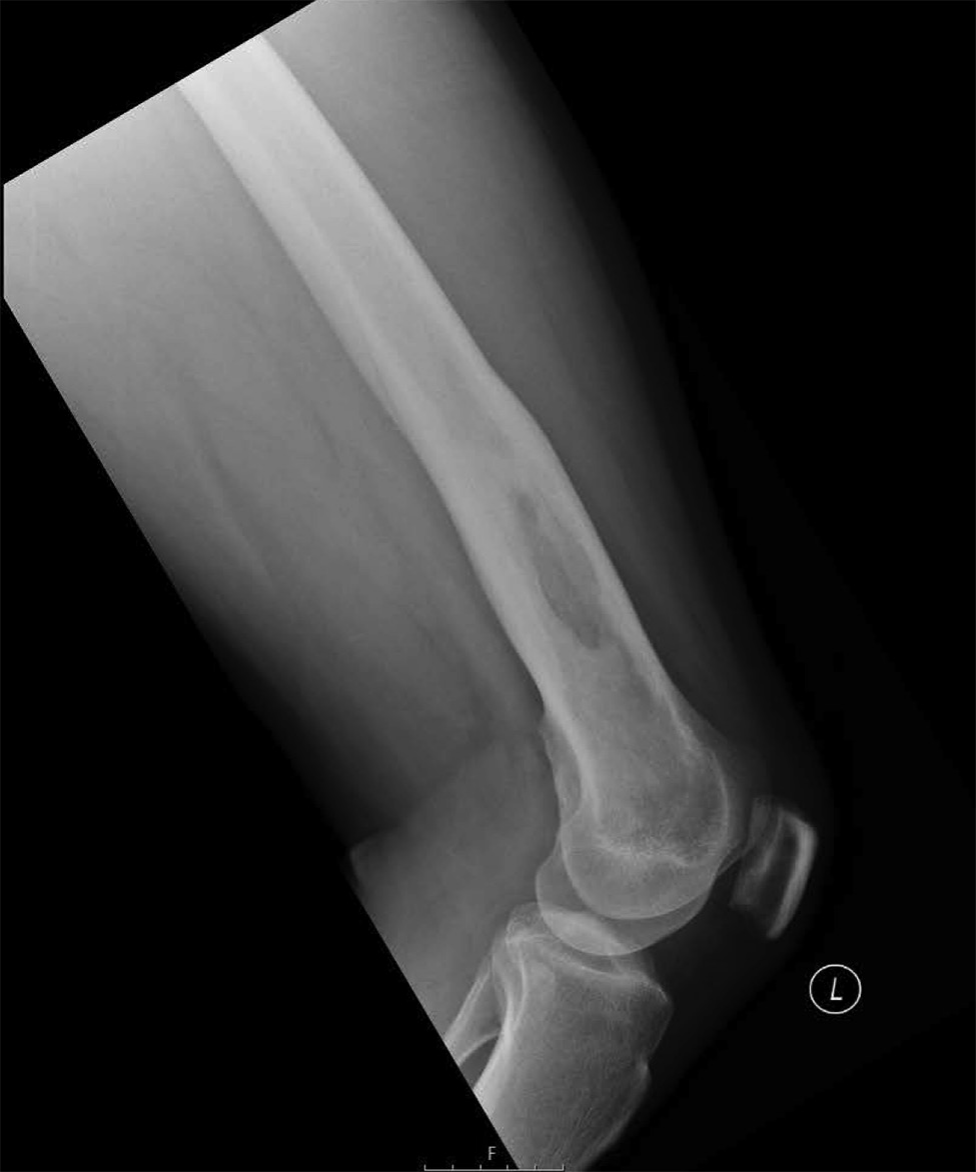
Follow-up radiograph for patient from Ghana with melioidosis manifesting as chronic femoral osteomyelitis of the left leg. Radiograph taken 12 months after initial assessment shows no remaining evidence of infection.
